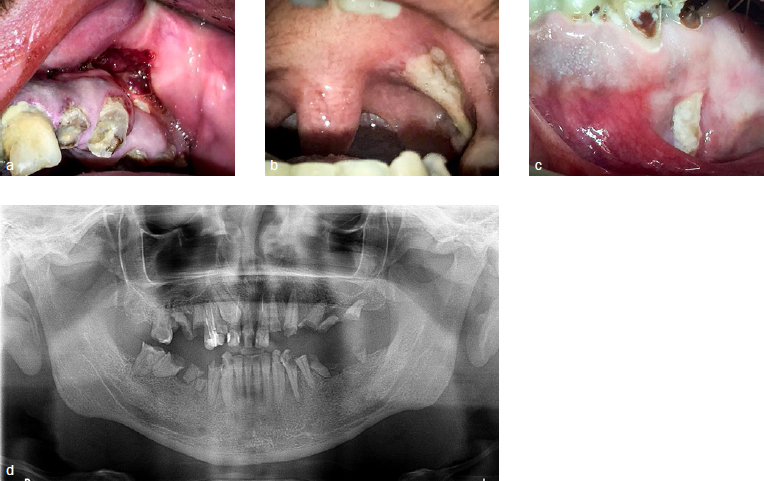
a) Ulcer with everted border in oral mucosa of the left maxilla; b) large ulcer in the left tonsillar pillar; c) mucosal fenestrations; d) initial panoramic radiograph.
